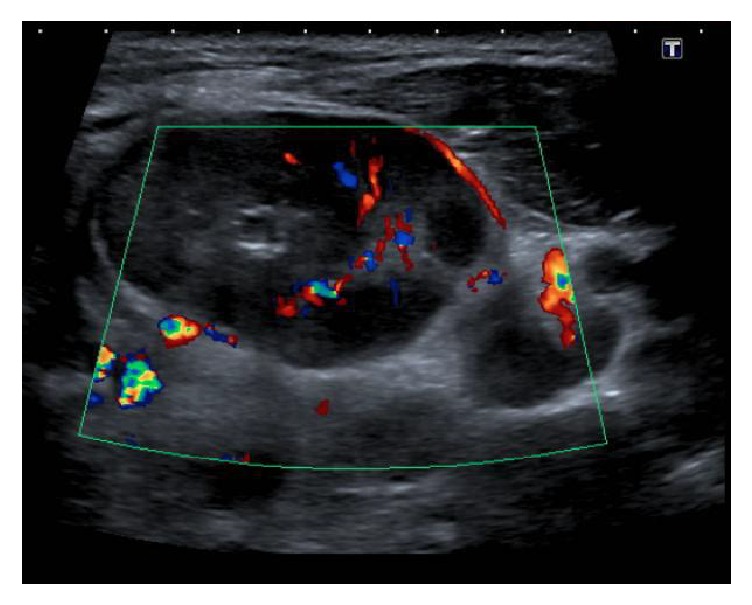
Ultrasound scan of the lesion in question showing colour Doppler flow in the posterior aspect of the lesion only.
